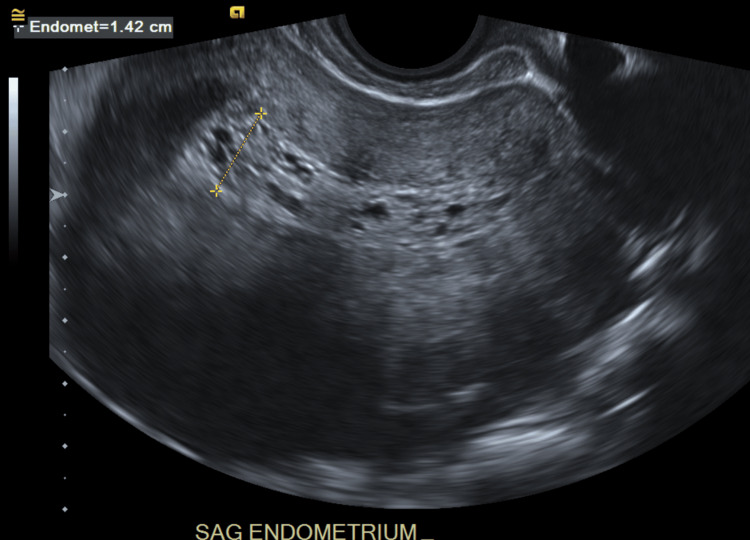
A transvaginal ultrasound sagittal view demonstrated endometrium thickening up to 14 mm.
